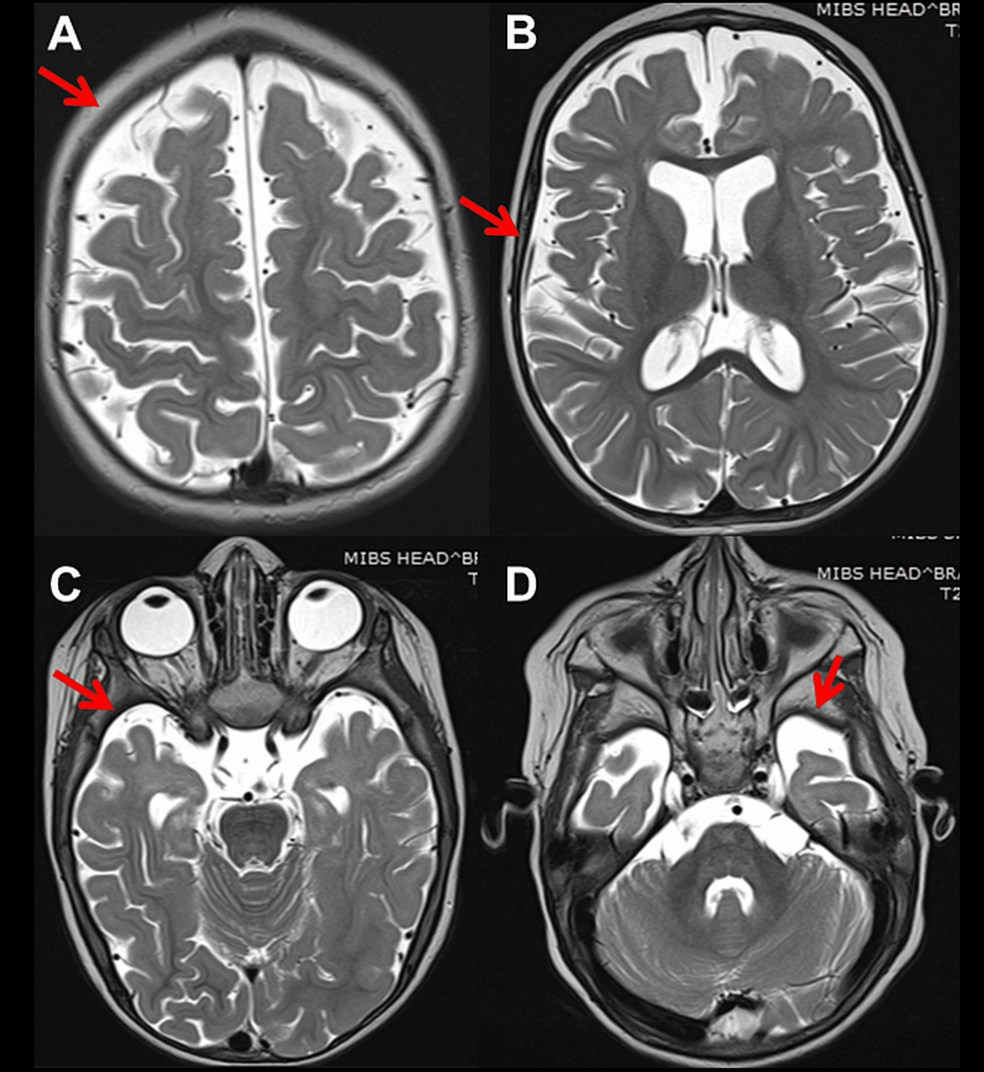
Cerebral MRI, T2-weighted images, at age 27 months showing hydrocephalus with dilation of lateral ventricles (B) due to reduced volume of the cortex (A), white matter, slight asymmetry of hippocampi (R > L), hyperintensities of the temporal poles bilaterally (C, D) and anterior parts of frontal lobes, smoothing of the cortico-medullary differentiation as a manifestation of incomplete myelination
